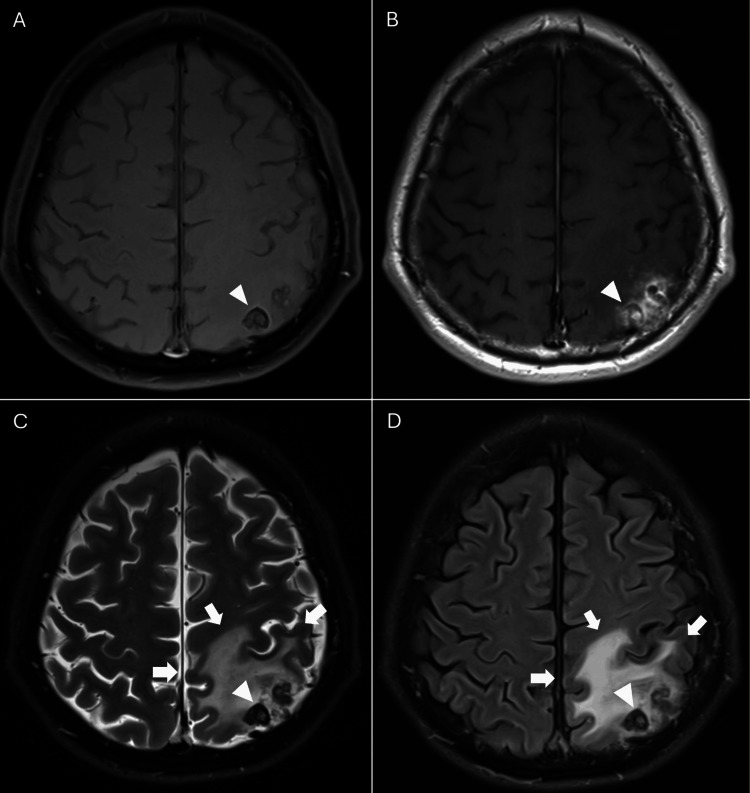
Cranial MRI obtained 13 years after stereotactic radiosurgery MRI: magnetic resonance imaging; FLAIR: fluid-attenuated inversion recovery Axial (A) T1-weighted image without contrast, (B) T1-weighted image with contrast, (C) T2-weighted image, and (D) FLAIR image reveal a small, round, heterogeneous lesion (with mixed iso-, hypo-, and mildly hyperintense signals) exhibiting a peripheral low-signal rim (arrowheads) and patchy internal and perilesional enhancement. Surrounding vasogenic edema (arrows) is noted, predominantly involving the left superior parietal lobule
